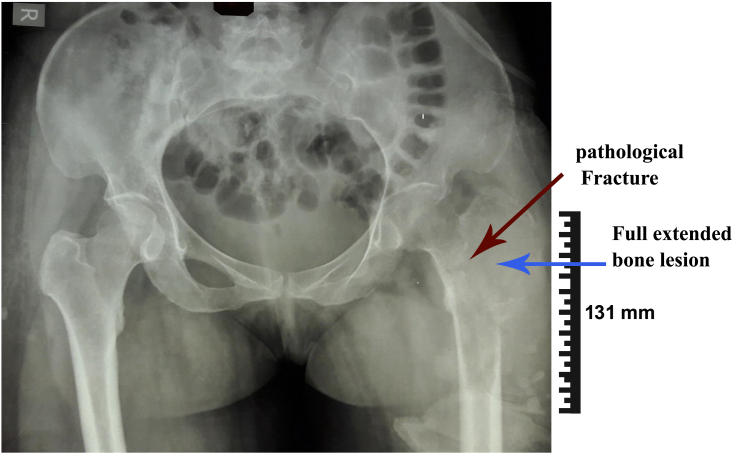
Plain radiograph of the pelvic demonstrated a pathological fracture of femur, large bone lesion and involvement of surrounded soft tissues in the presented patient.
